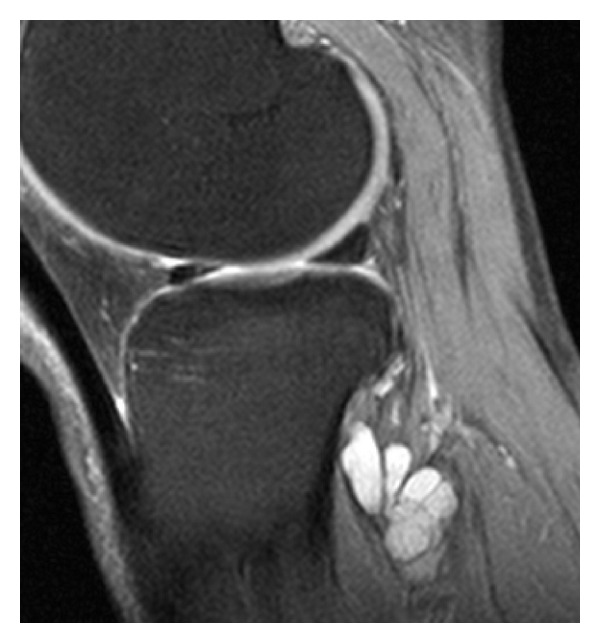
Multiple cysts within the popliteus muscle. Sagittal proton fat-saturated MR image of the right knee without effusion or synovitis in a 56-year-old male floor layer. The lesions represent an abnormal extension of the popliteus tendon synovial recess.
